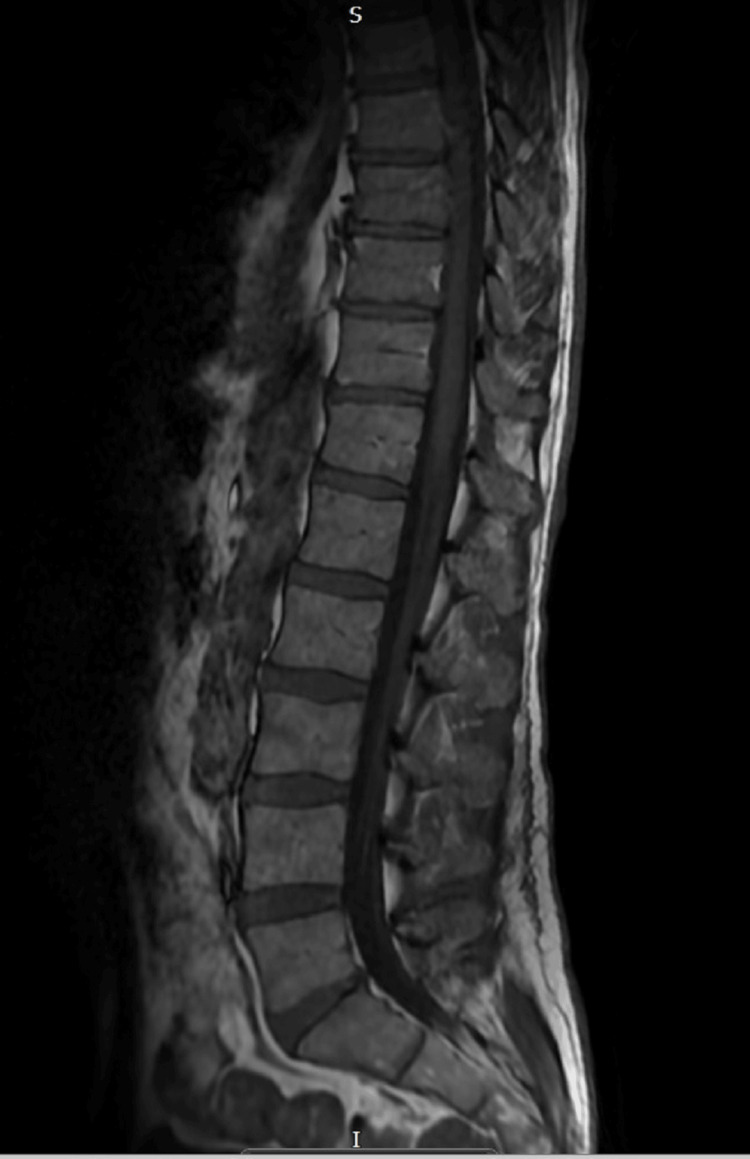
Normal MRI spine Unremarkable appearances of the cord, conus, and cauda equina. MRI: magnetic resonance imaging
